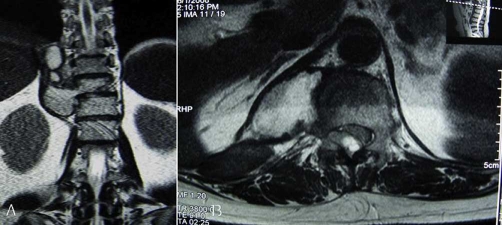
(A) Coronal MR images showed paravertebral lesion had expanded to the T11 neural foramina. (B) Axial T2-weighted MR images revealed spinal cord was compressed due to the multilocular hydatid cyst extended into the spinal canal. The main part of the lesions was located at the right side of T11.
